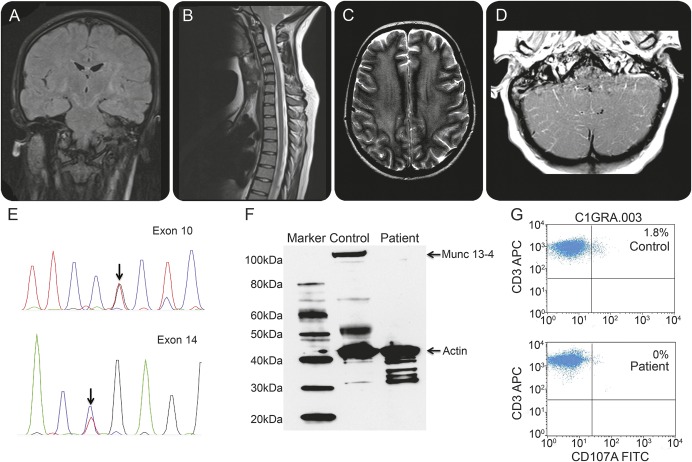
Imaging findings, Sanger sequencing, Munc protein expression, and CD107a degranulation assay in a 14-year-old female with progressive neurologic disorder due to primary hemophagocytic lymphohistiocytosis (A) Coronal fluid-attenuated inversion recovery–weighted MRI of the brain, (B) sagittal T2-weighted imaging of the cervicothoracic spine, and (C) axial T2-weighted imaging of the brain, showing bilateral lesions in the brain (internal capsules, globi pallidi, subthalamic nuclei, deep white matter), as well as mid–cervical spine lesions. (D) Axial postcontrast T1-weighted imaging at the level of the cerebellum revealed bilateral diffuse leptomeningeal enhancement. (E) Sanger sequencing chromatogram of *UNC13D* gene showing compound heterozygous mutations in exon 10 (c.C817T; p.R273X) and exon 14 (c.G1241T; p.R414L) for the same patient. (F) Western blot analysis in patient-derived peripheral blood mononuclear cells shows absence of Munc 13-4 protein relative to a healthy control. (G) Flow cytometric figures show absent percentage increase in CD107a-positive cells in patient-derived CD8 T cells following stimulation with anti-CD3 antibody in relation to a healthy control.
